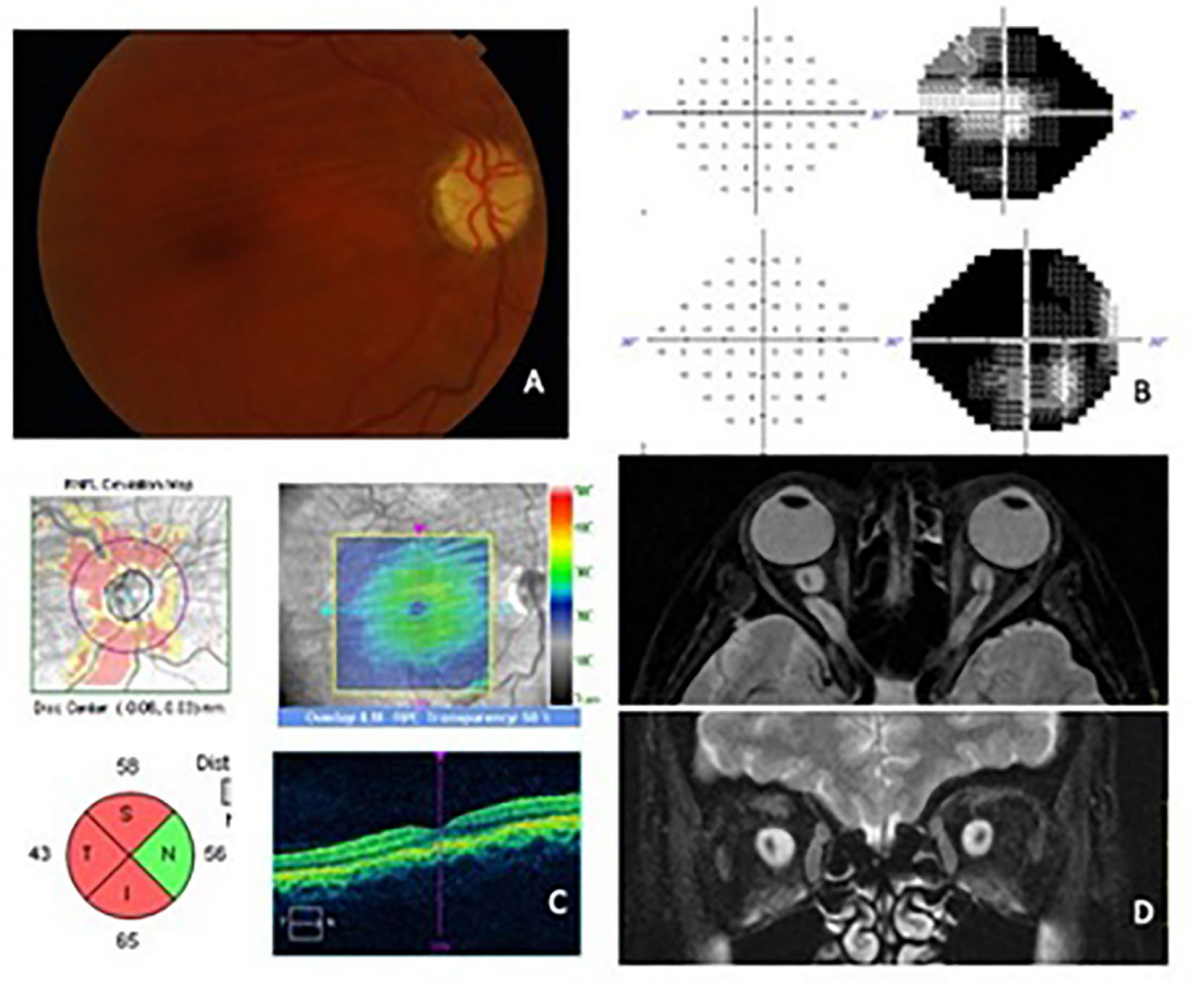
Case 1 (images after bilateral ONSF). (A) Right eye fundoscopy showing choroidal folds and optic nerve pallor. (B) 24-2 Visual field of the left eye and the right eye. A concentric contraction of the visual fields, more pronounced in the right eye, is shown. (C) ONH and macular OCT scans of the right eye with RNFL layer atrophy and choroidal folds in the posterior pole. (D) Orbital MRI. Axial and coronal view in T2 with fat suppression showing the marked dilatation of optic nerve sheath in both eyes.
